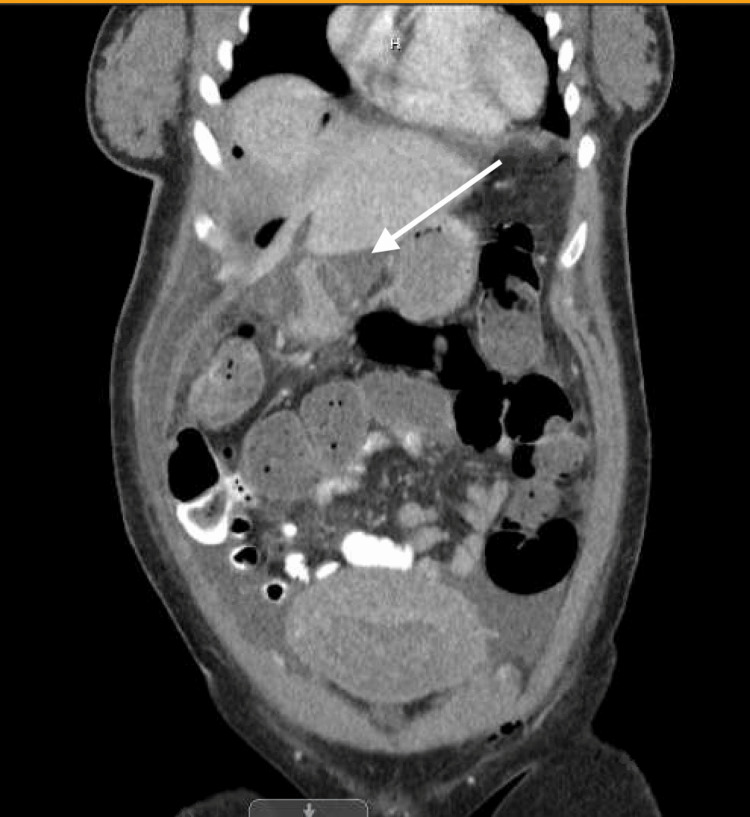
Contrast-enhanced CT abdomen and pelvis (coronal view). The white arrow indicates a fluid-containing structure along the anterior abdominal wall, which may represent bowel injury versus a developing postoperative fluid collection or abscess.
